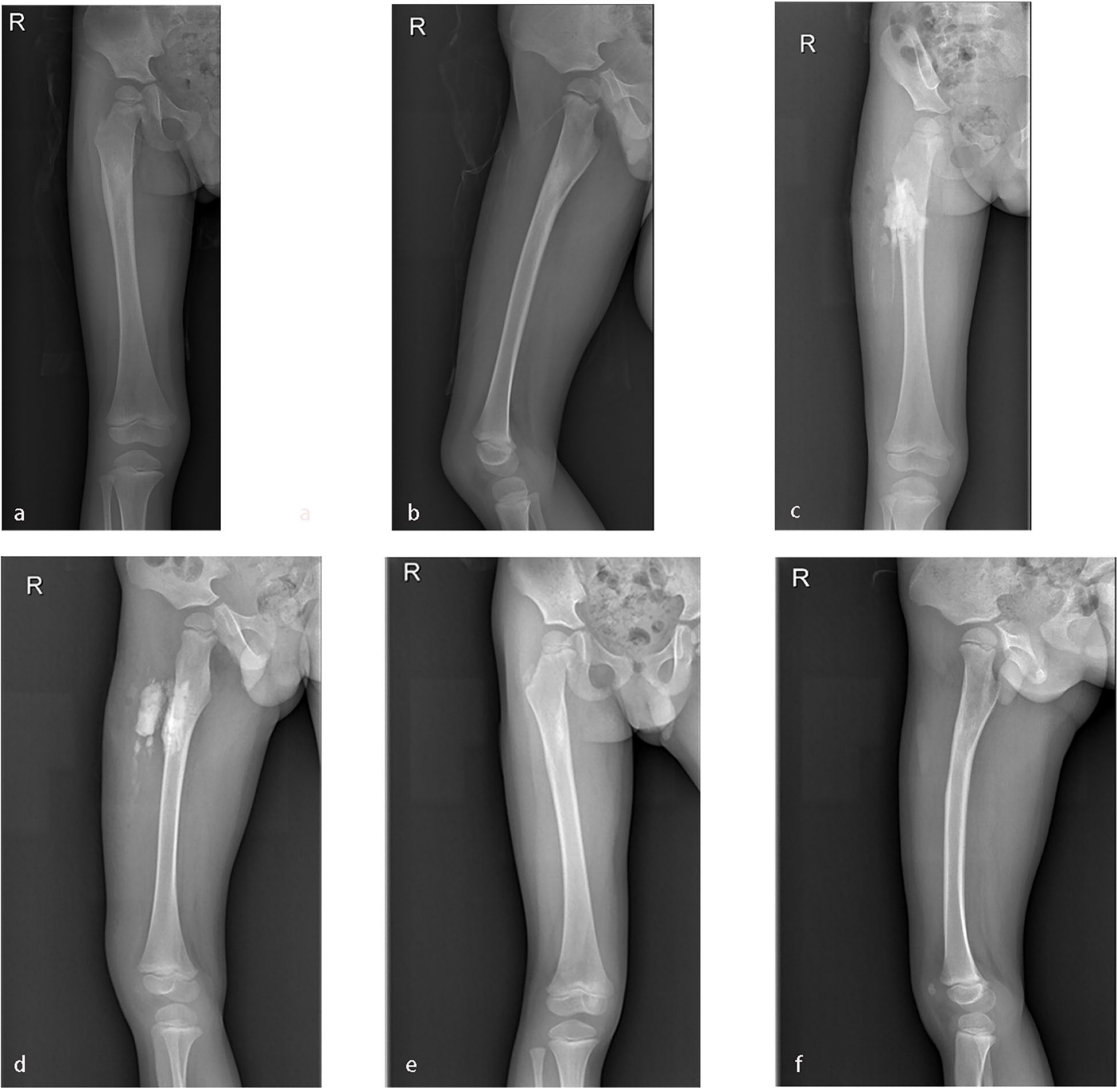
a-b: A three-year-old boy suffered right proximal femoral HO. c-d: Vancomycin impregnated CS was used to fill the void left by decompression and debridement. e–f: X-ray examination indicated good recovery 12 months postoperatively
